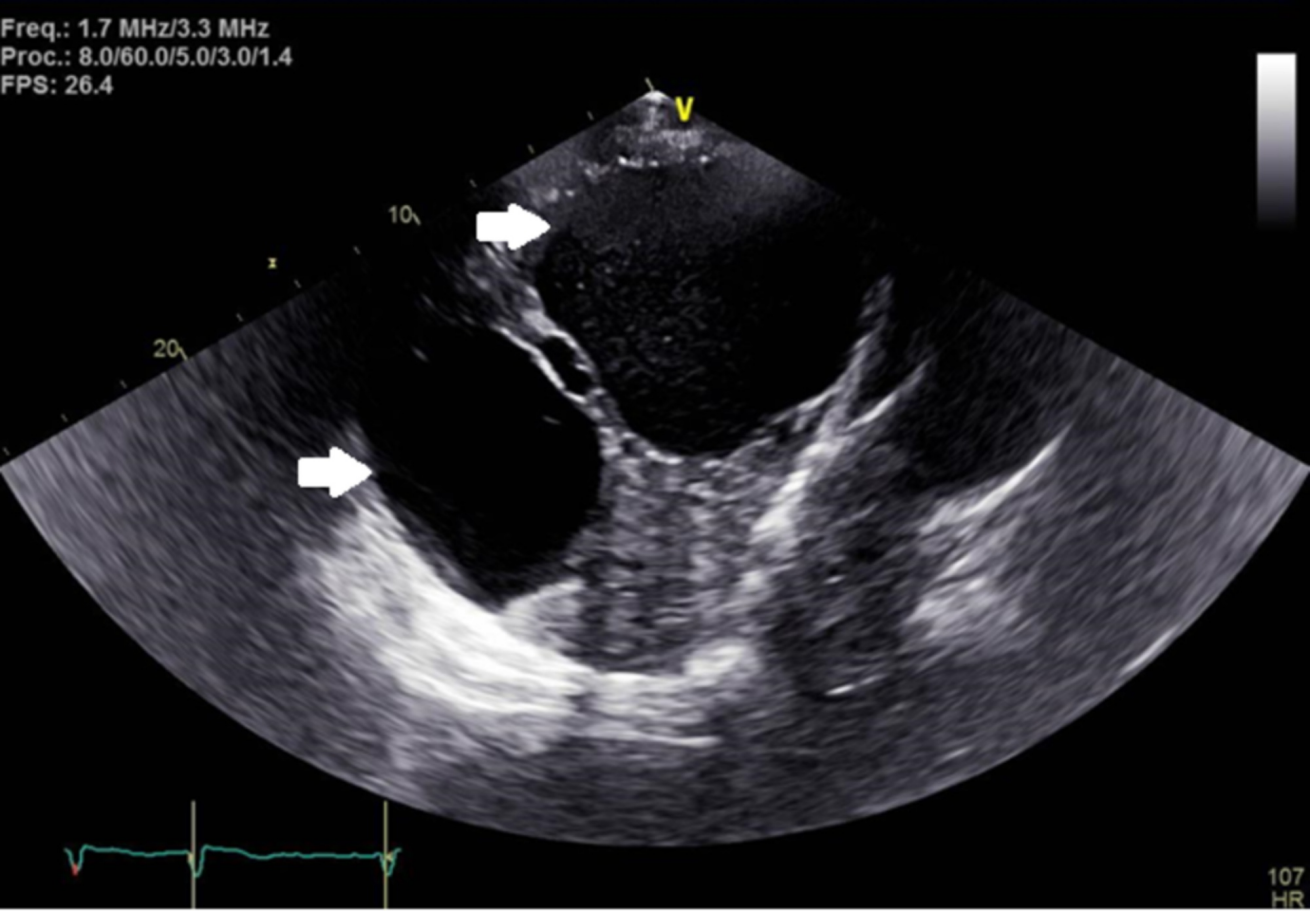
Transthoracic echocardiograph with a subxiphoid view, with arrows indicating liver cysts
